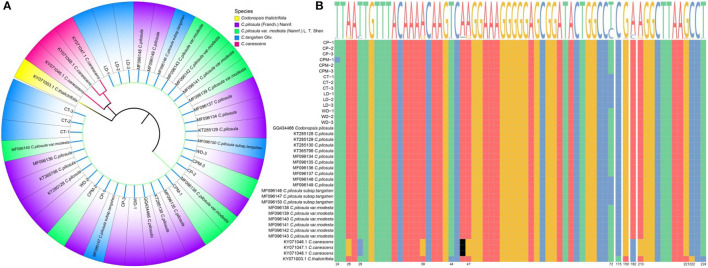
Construction of NJ tree of Codonopsis Radix samples based on ITS2 sequences (A). ITS2 sequence variant site information (B). LD, WD, CP, CPM, and CT reported Luodang, Weidang, *C. pilosula, C. pilosula* var. *modesta*, and *C. tangshen*, the same below.
